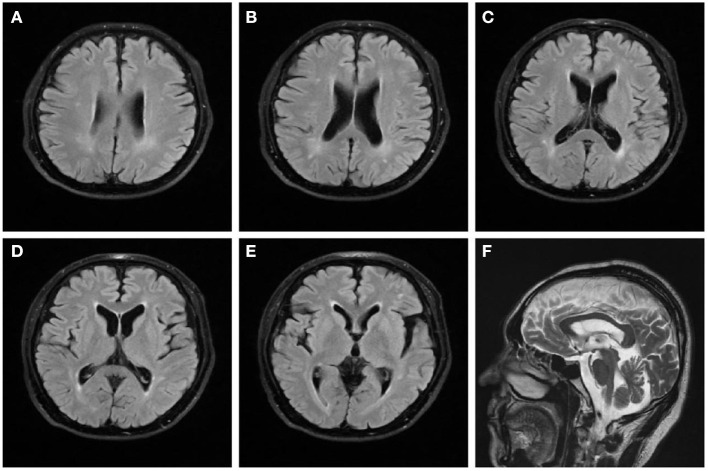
Brain magnetic resonance imaging of the patient. FLAIR sequences (A–E) reveal high signal changes in the periventricular white matter and mild brain atrophy. T2-weighted (F) sequence shows that the corpus callosum is normal.
